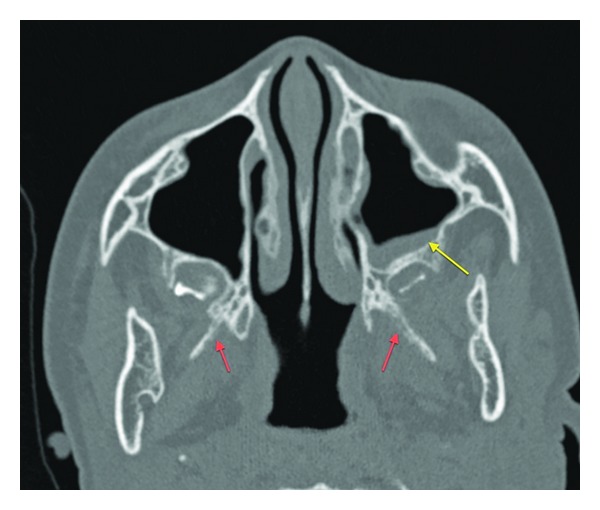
Axial CT: mucosal thickening of the left maxillary antrum is noted with mural bony thickening (yellow arrow). There are erosive changes involving the lateral pterygoid plates (red arrows).
